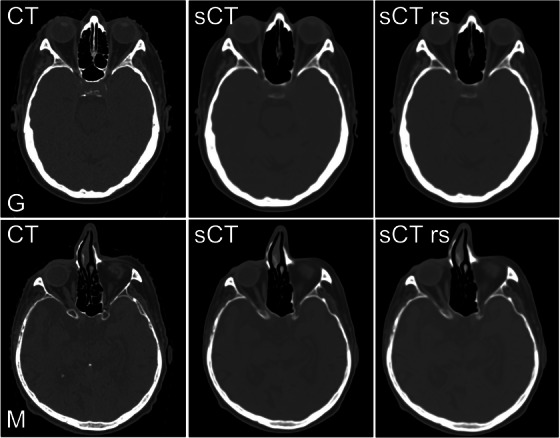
Image quality comparison between the CT, sCT, and resampled sCT (sCT rs) images used during resampling effect evaluation. Images for the outlier glioma patient are shown in the upper row. Images for the outlier metastasis are shown in the bottom row. Images are presented with identical windowing settings. CT, computed tomography; G, glioma; M, metastasis; rs, resampled; sCT, synthetic computed tomography.
